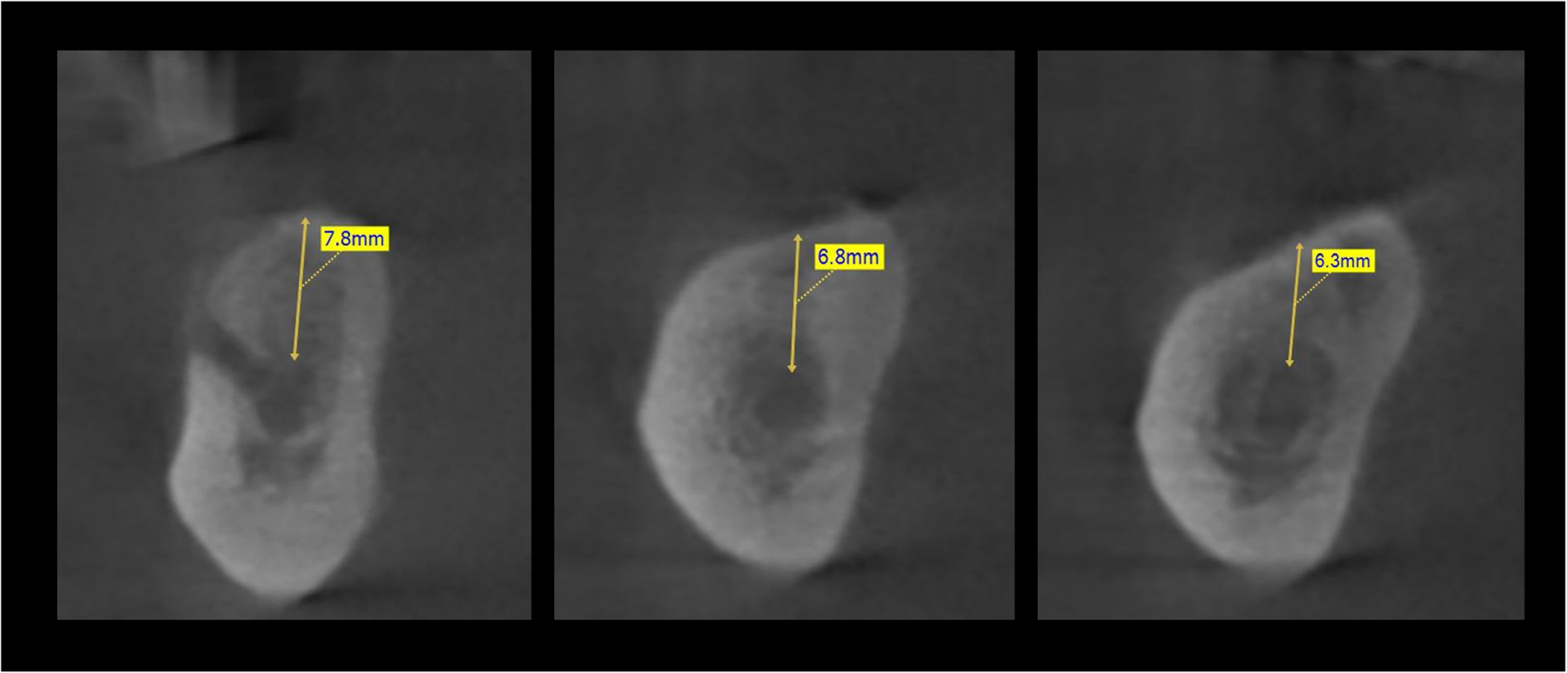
A CT scan before surgery
